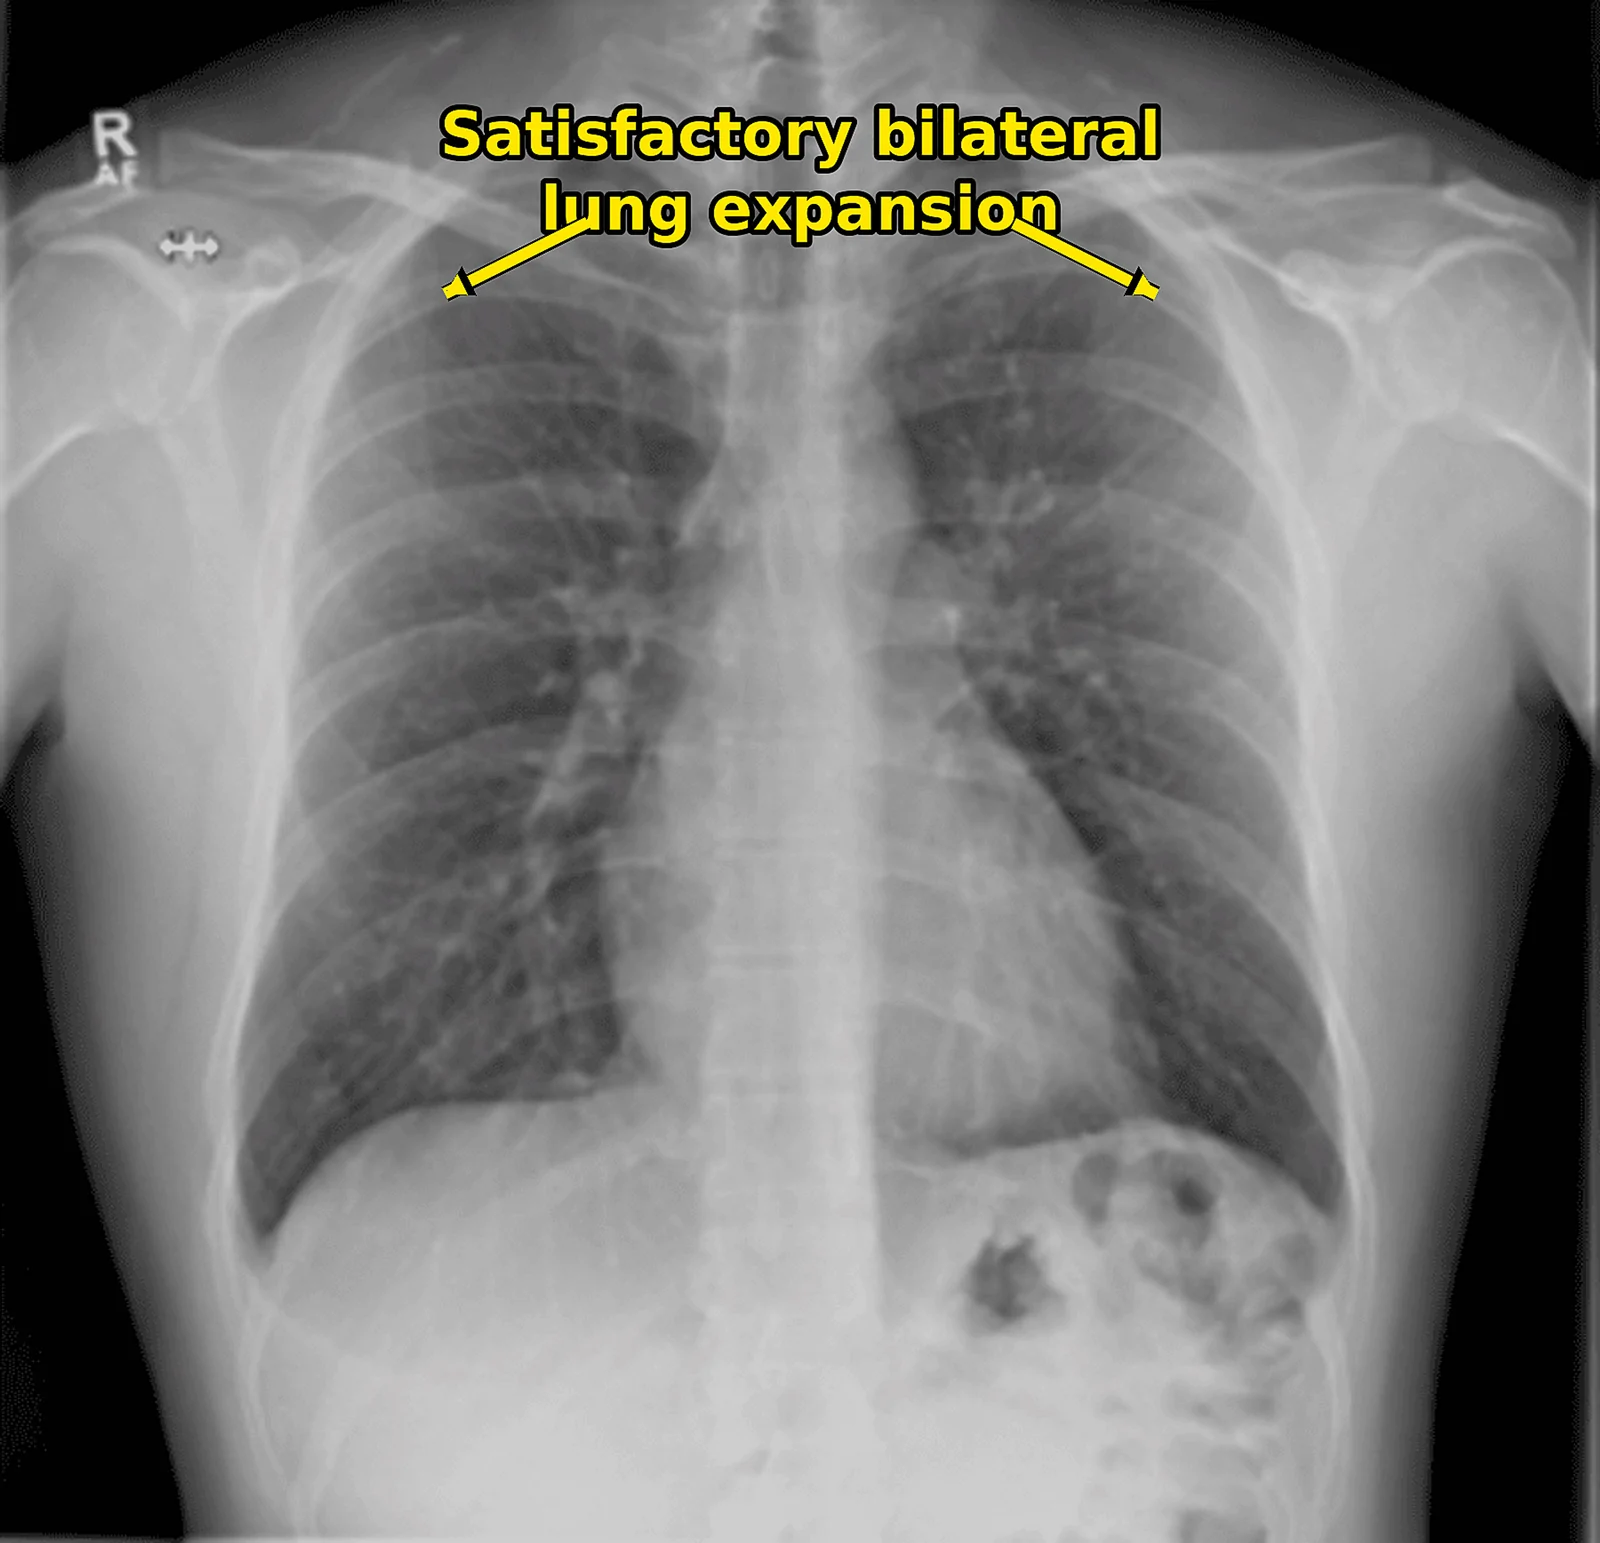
Follow-up chest radiograph obtained eight hours after drain removal: satisfactory bilateral lung expansion without immediate radiographic recurrence
